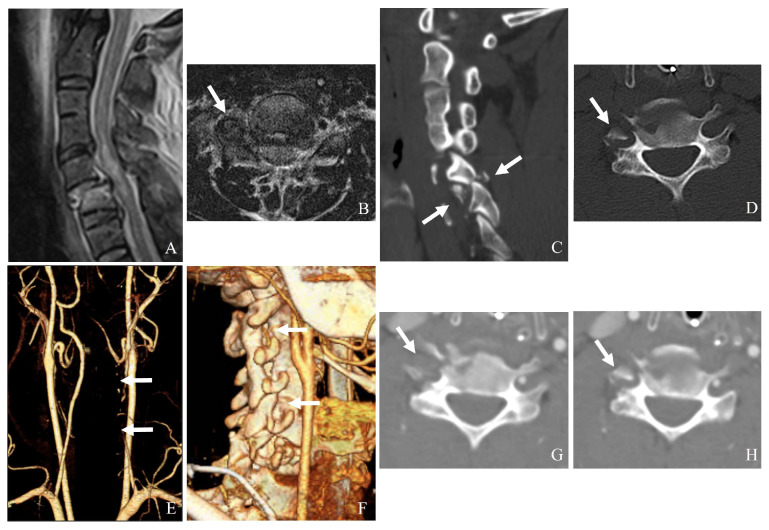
Magnetic resonance imaging (A,B) and computed tomography (C,D) demonstrate C5–C6 bilateral facet dislocation, traumatic disc herniation, and a right transforaminal fracture fragment (white arrows). Neck computed tomography angiography (E–H) reveals a right vertebral artery injury caused by the transforaminal fracture fragment (white arrows).
